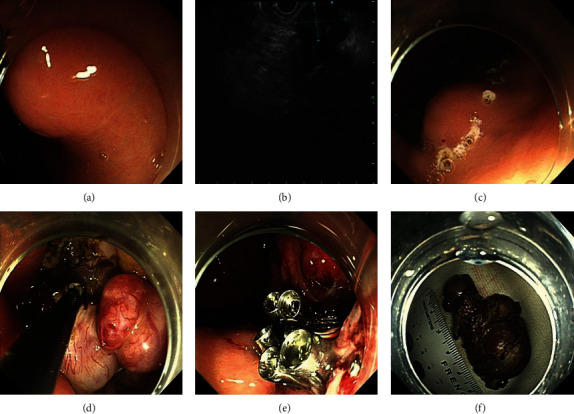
Endoscopic full-thickness resection treatment for a gastric GIST: (a) gastric GIST identified by endoscopy; (b) the same gastric GIST observed by endoscopic ultrasound; (c) marking outside the tumor; (d) resection of the tumor; (e) close the wound; (f) the resected tumor. GIST: gastrointestinal stromal tumor.
